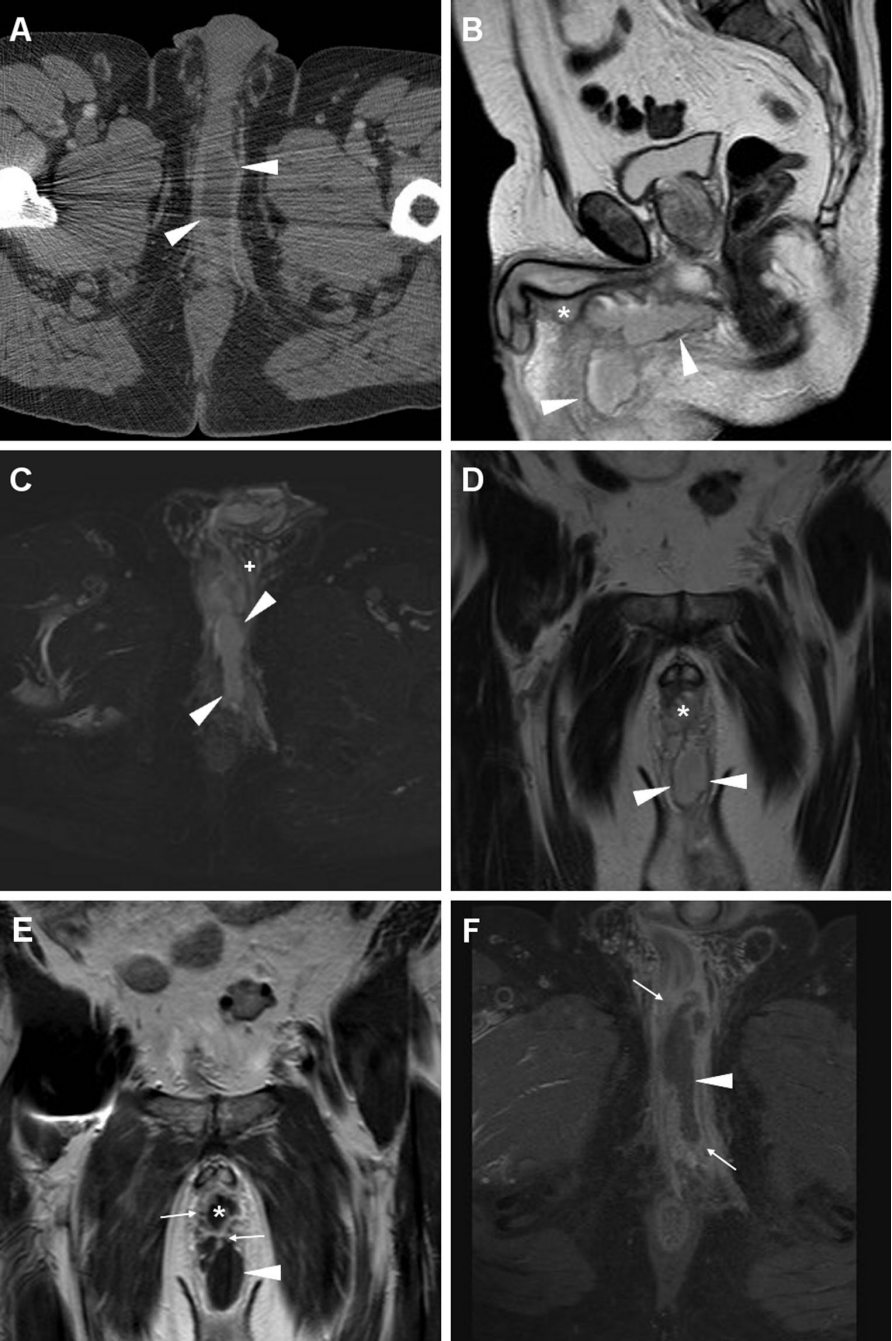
Urethral infection complicated by penile and perineal abscess in a 53-year-old man with tender, inflamed perineal swelling despite antibiotics. Infection was initially detected at contrast-enhanced CT (a) as an elongated midline abscess with peripheral enhancement (*arrowheads*) and internal fluid. MRI showed corresponding inhomogeneous fluid-like content on T2-weighted sequences (b–d), with surrounding inflammatory stranding (+) and strong contrast enhancement in the abscess walls (*arrowheads* in e, f). The infected corpus spongiosum (*) showed similar signal features. Surgical evacuation was required to relieve the abscess
